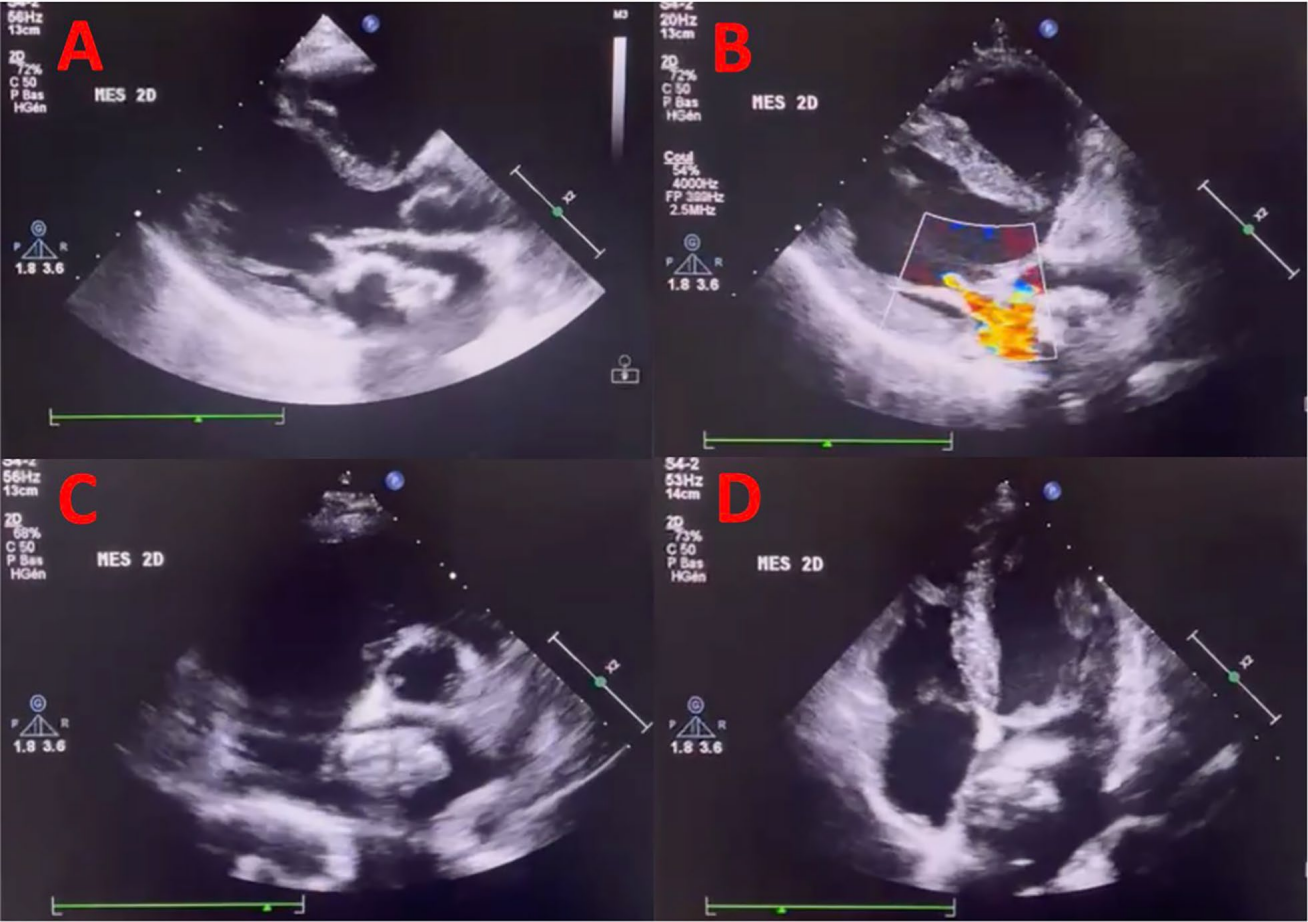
Transthoracic echocardiogram showing the left atrial mass. A Parasternal long axis showing the mass attached to the mitral valve. B Parasternal long axis showing mitral regurgitation. C Parasternal short axis showing the mass attached to the atrial septum. D Apical 4 chamber view showing the mass mimicking an atrial myxoma
